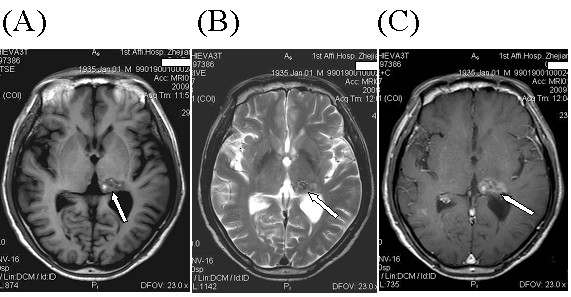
Case 2. A: T1-weighted brain MRI shows a metastatic lesion of heterogeneous signal intensities in the left thalamus (arrow). Components of hyper-signal intensities represent subacute hemorrhage. B: This lesion is also of heterogeneous signal intensities on T2-weighted images, with components of hypointense hemosiderins representing chronic hemorrhage (arrow). C: Contrast-enhanced MRI shows inhomogeneous enhancement of the lesion (arrow).
